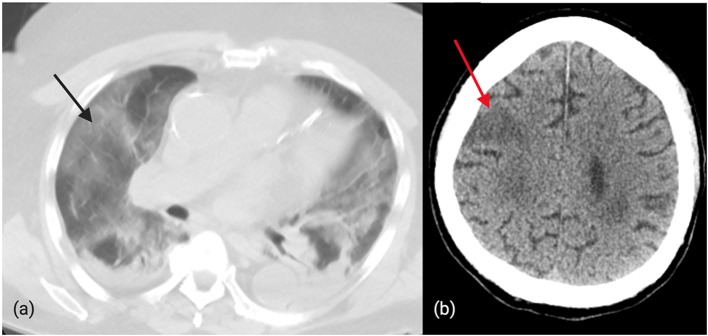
(a): Diffuse, bilateral ground-glass opacities in a patient with confirmed COVID-19 and distal MCA occlusion. (b): CT brain revealing ischemic changes in the right cortical MCA territory in a patient with COVID-19.
